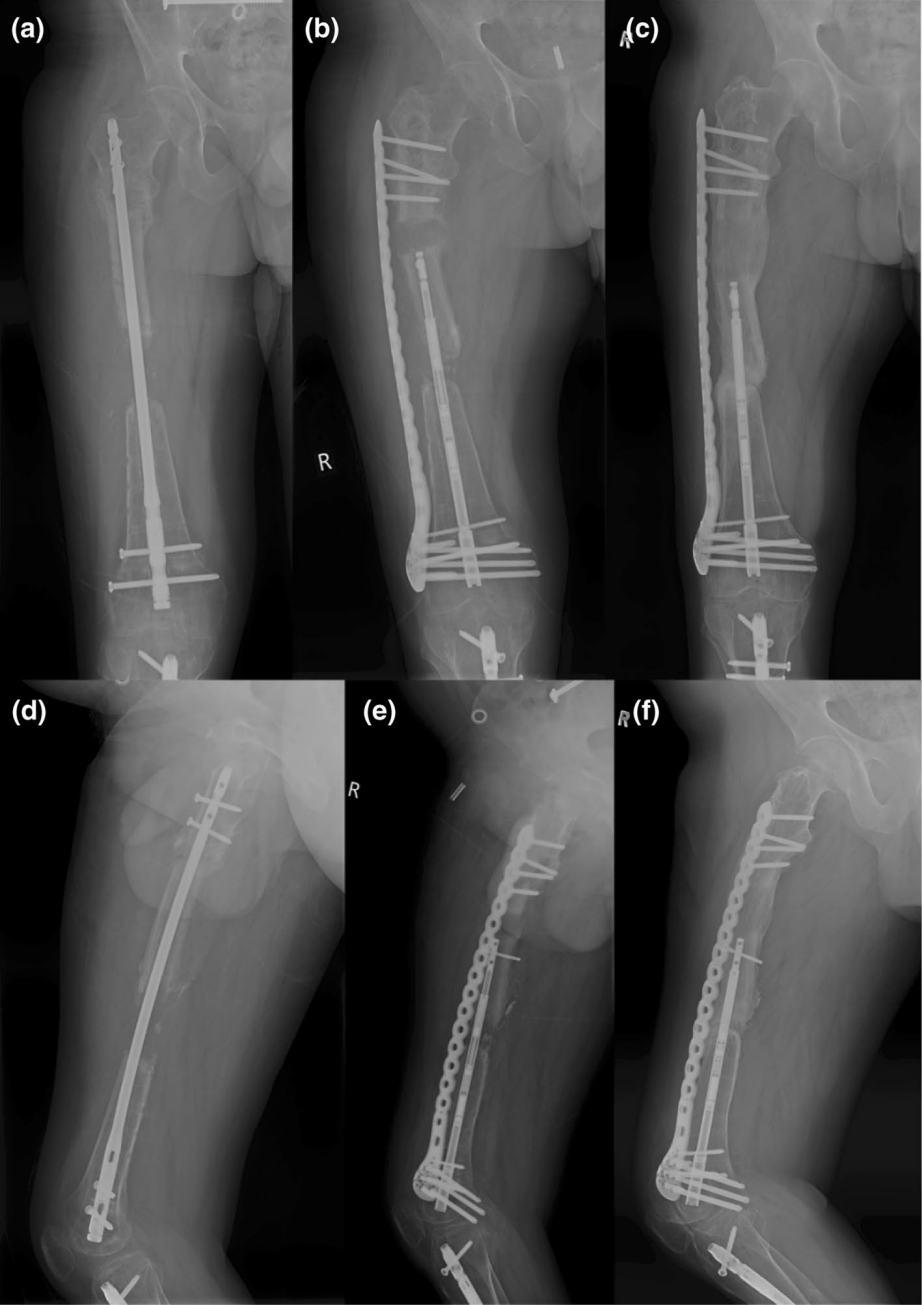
AP (a, b, c) and lateral (d, e, f) radiographs of Patient 1 showing the defect at the start, during the retrograde bone transport and at the final stage. Patient 1 had a previously unsuccessful bone transport by MRS Ex-Fix before using magnetic lengthening nails
